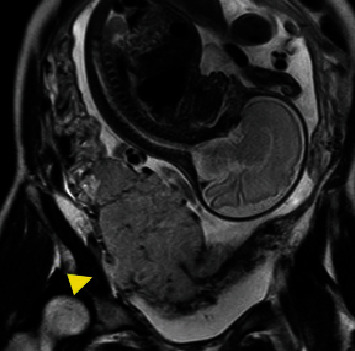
MRI T2 coronal section. The muscle layer on the right side of the lower uterine corpus appears to be replaced by the placenta (yellow triangle), but a ureteral invasion is not suspected. MRI, magnetic resonance imaging.
